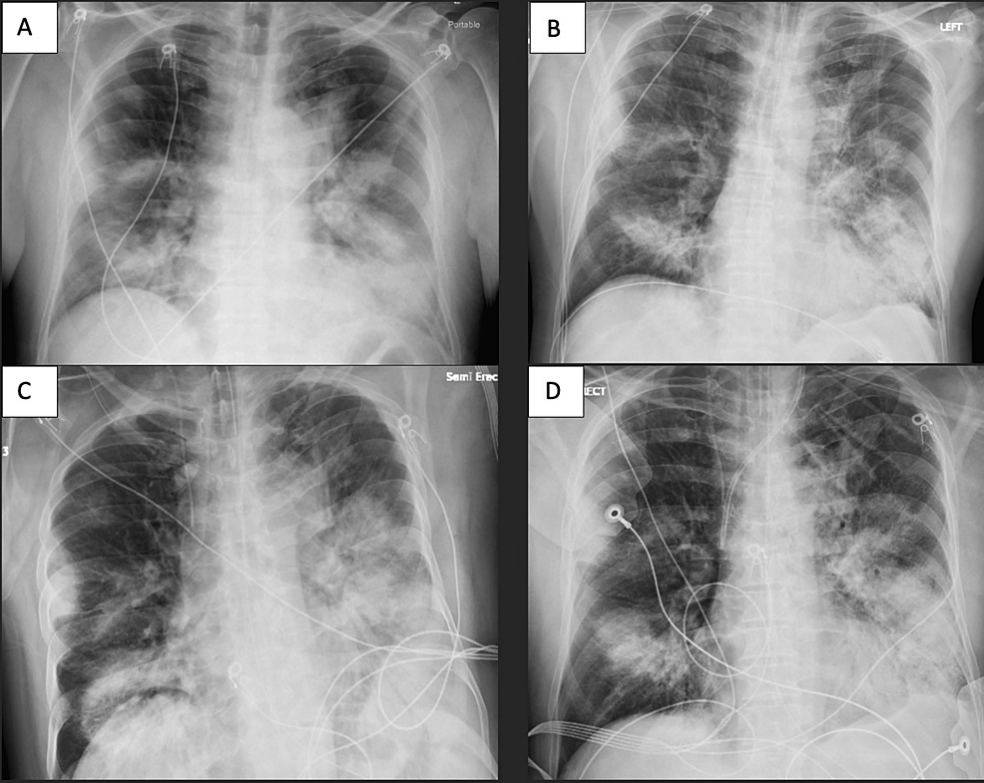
Progressive pulmonary infiltration in the setting of Legionnaire’s disease (A) Initial chest X-ray on hospital day (HD) 0 demonstrating bilateral, poorly-delineated consolidative airspace opacifications; (B) HD 1 following intubation for acute hypoxic respiratory failure – re-demonstration of consolidative bilateral multifocal airspace opacification; (C) HD 2 interval placement of a right internal jugular central venous catheter due to persistent hypotension requiring vasopressor support with X-ray demonstrating worsening infiltration versus mild pulmonary edema; (D) HD 3 demonstrating interval insertion of a left-sided Quinton catheter due to anuric renal failure and interval evolution of possible left lower pleural effusion and predominant opacification in the left mid and lower lung as well as the right lower lung.
